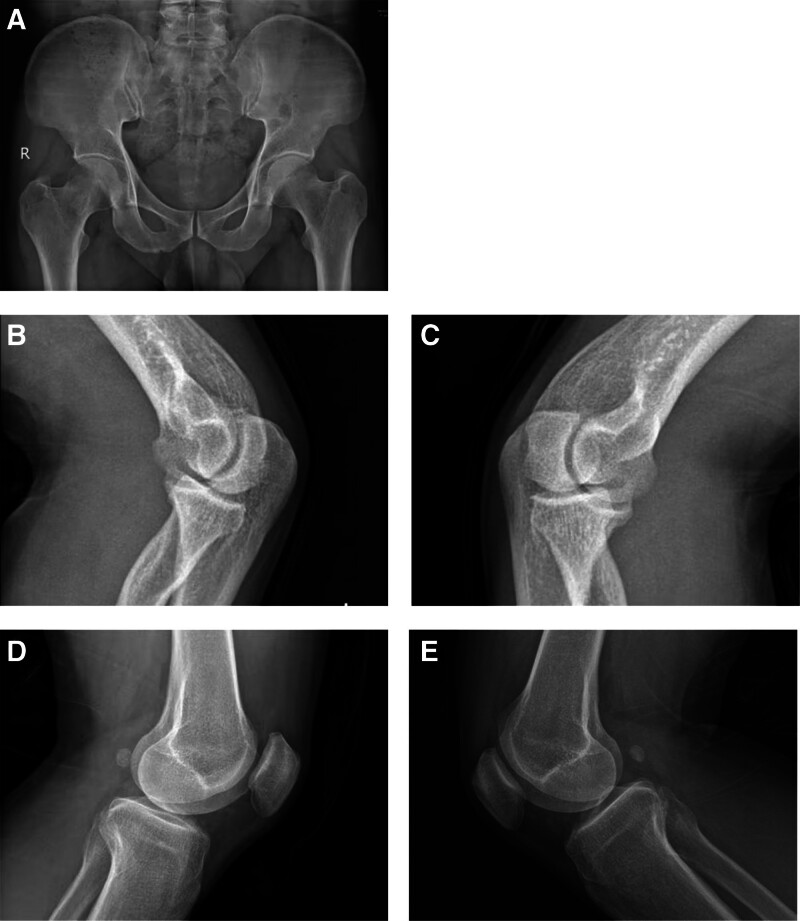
Radiographs of the pelis, elbows, and knees of the uncle (II:4), A is the pelvis of patient II: 4, B and C are the left and right elbow joints of patient II: 4, and D and E are the left and right knee joints of patient II: 4, respectively.
